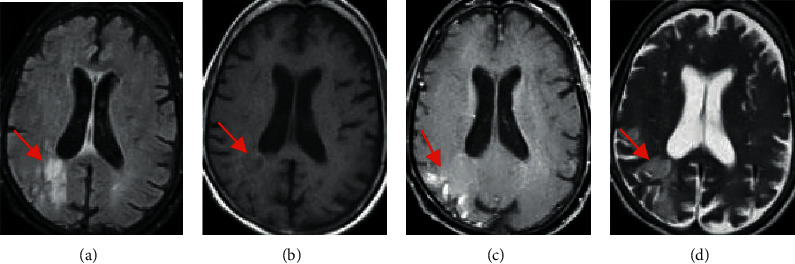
MRI image of a 58-year-old female patient. The arrows in the images indicate the relevant stroke area.
